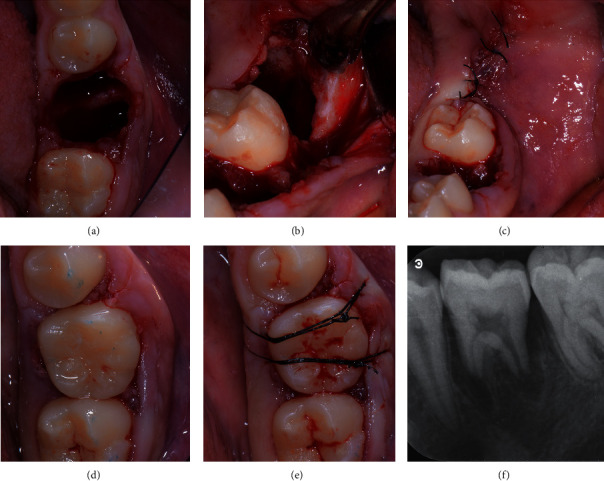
Tooth 36: (a) alveolar extraction socket; (b) alveolar extraction socket of left third molar; (c) sutures of third molar extraction site; (d) transplanted tooth in position 36; (e) cross-sutures for stabilization; (f) periapical radiograph immediately after transplantation.
